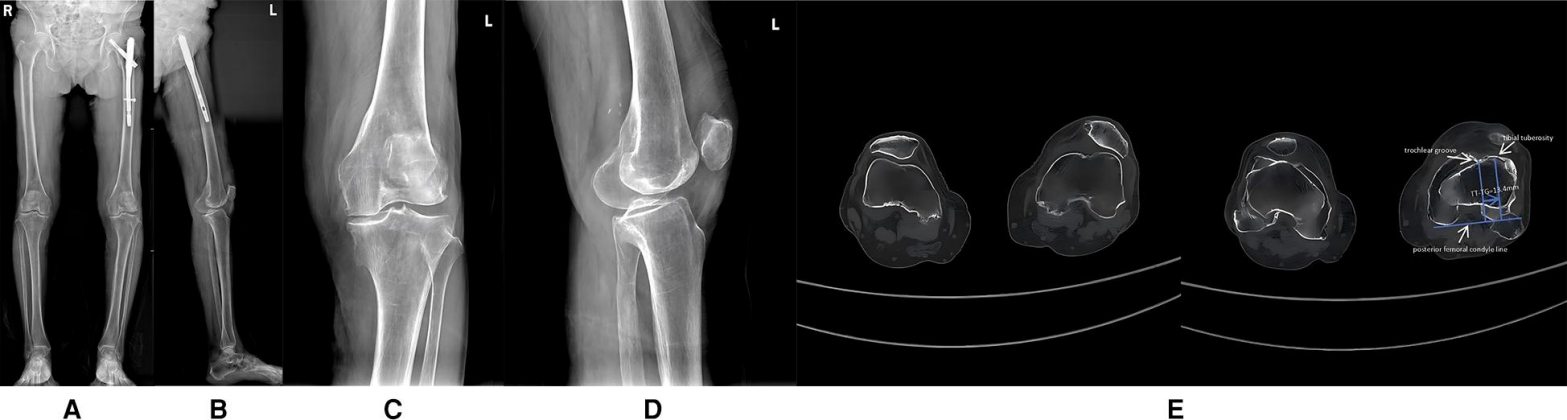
(A) Full-length frontal radiograph of the lower limb; (B) Full-length lateral radiograph of the lower limb; (C) Frontal radiograph of left knee joint; (D) Lateral radiograph of left knee joint; (E) CT axial images of left knee joint. [The tibial tuberosity–trochlear groove (TT-TG) distance is 13.4 mm].
